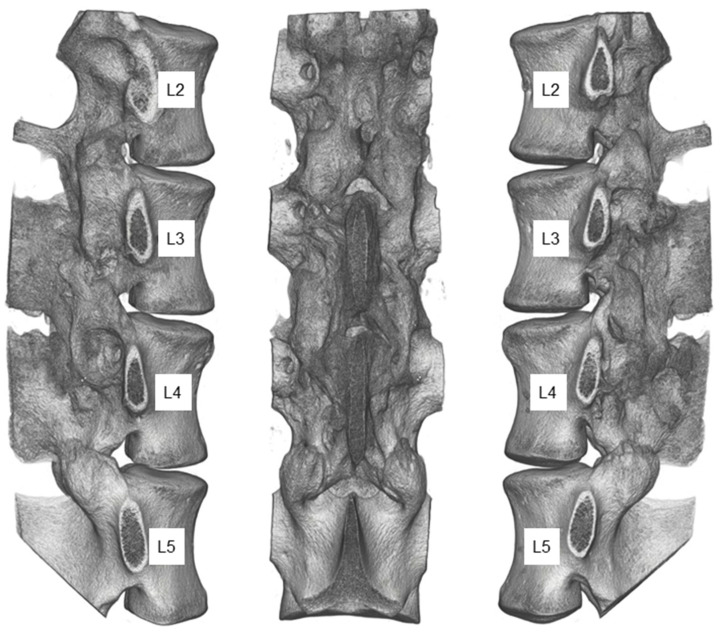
The lumbar spine’s lateral and posterior views (L2–L5) after euthanasia and implant removal derived from high-resolution CT data.
